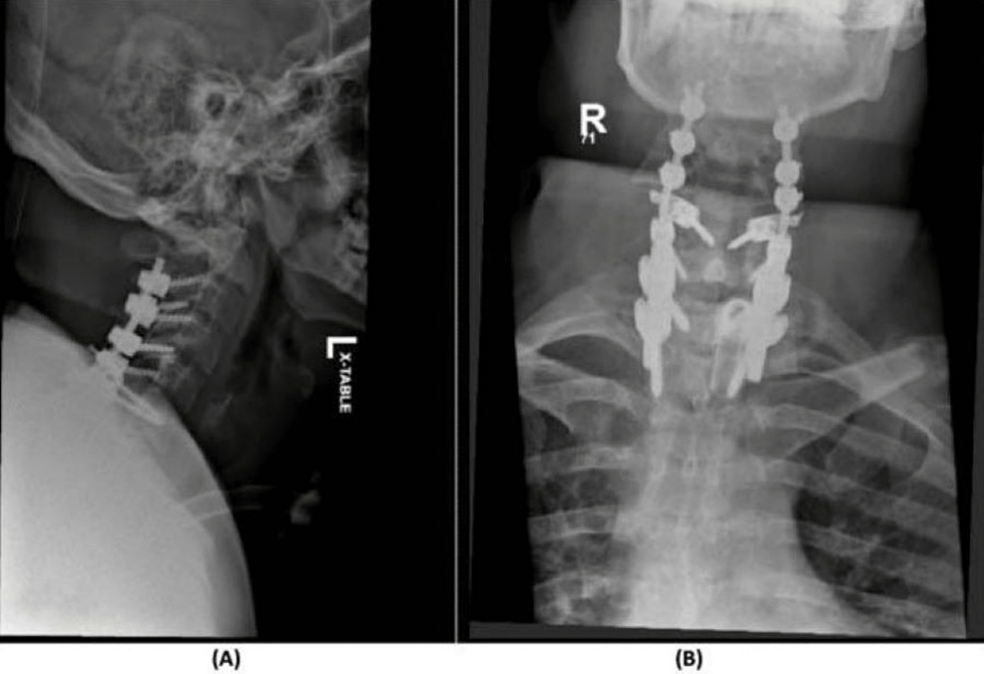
X-rays of final operative reduction and fixation (A) Lateral cervical spine X-ray demonstrating final fixation from C3–T2; (B) AP spine X-ray demonstrating the corresponding level.
